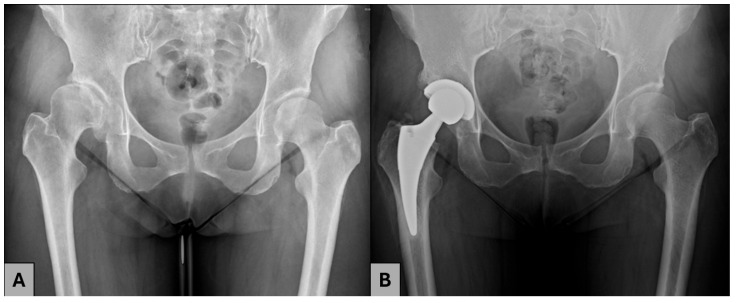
A 44-year-old female patient with residual DDH. (A) AO radiograph before THA; (B) AP radiograph after THA.
